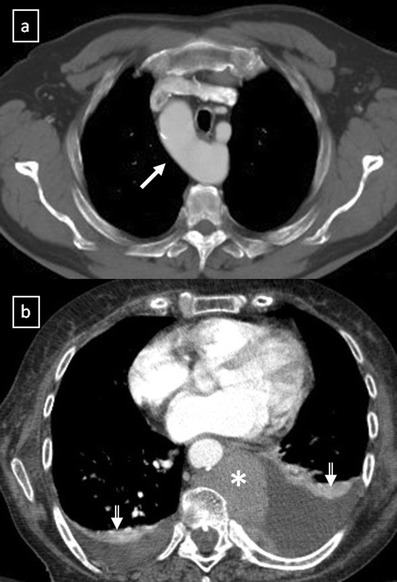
a Contrast-enhanced CT scan shows a right-sided aortic arch (*arrow*) in an asymptomatic man with an absence of the aortic knuckle on the left. b Contrast-enhanced CT scan demonstrates a soft tissue posterior mediastinal mass (*) in a 66-year-old woman with multiple myeloma diagnosis. Note the mass effect to the descending aorta and left auricula by the mass and the bilateral pleural effusion (*open arrows*)
